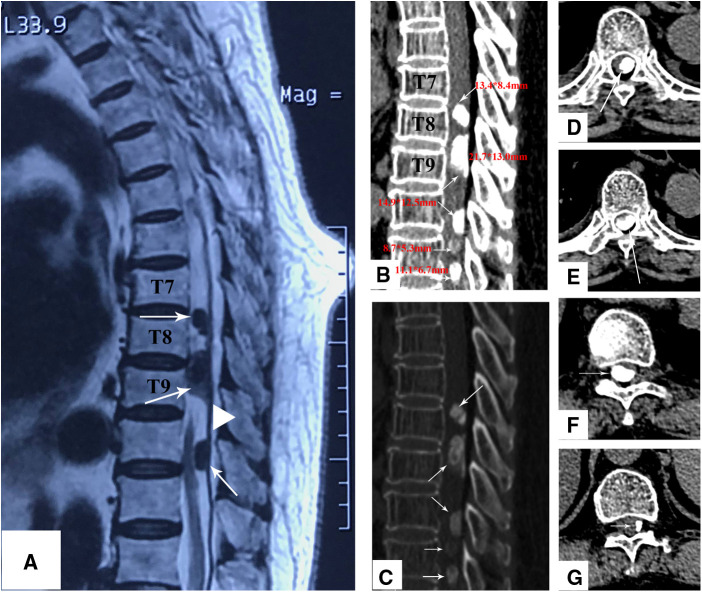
(A) Sagittal T2-weighted MRI shows three large lesions at the T7–T11 level with low signal intensity. The lesions shown with a dural tail sign. (B,C) The sagittal CT scan shows that there are five different sizes of high-density masses at the T7–T8 (D), T8–T9 (E), T10–T11 (F), and T11–T12 (G), levels, and all of them are located at the posterior of the spinal canal.
